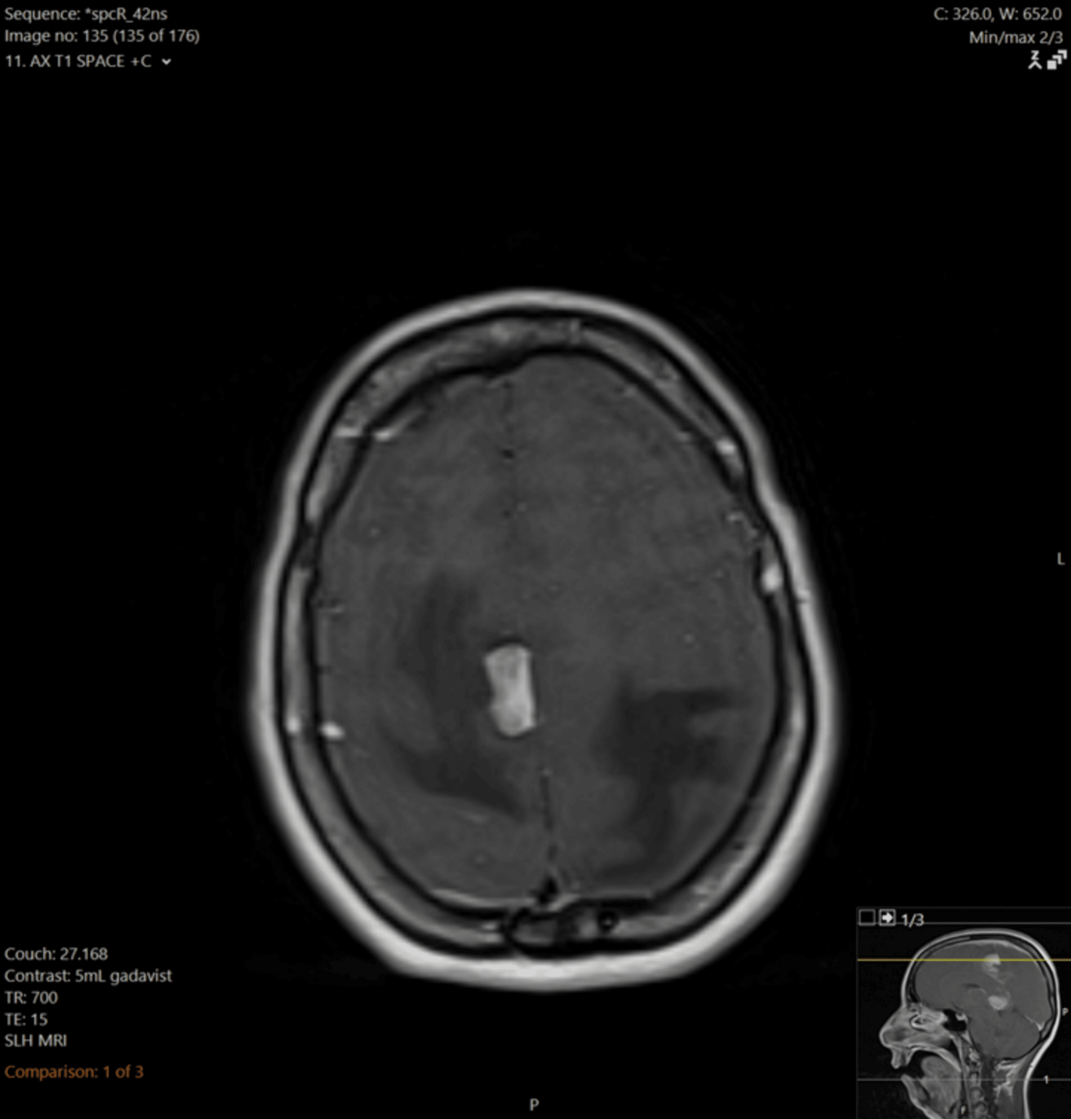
T1 post-contrast MRI image with interval enlargement of the right frontoparietal lesion with adjacent vasogenic edema MRI: magnetic resonance imaging
